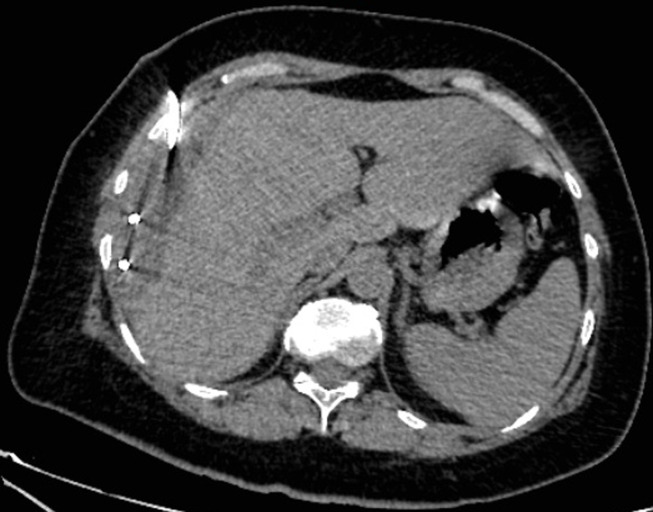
CT-scan at the first day after drainage
